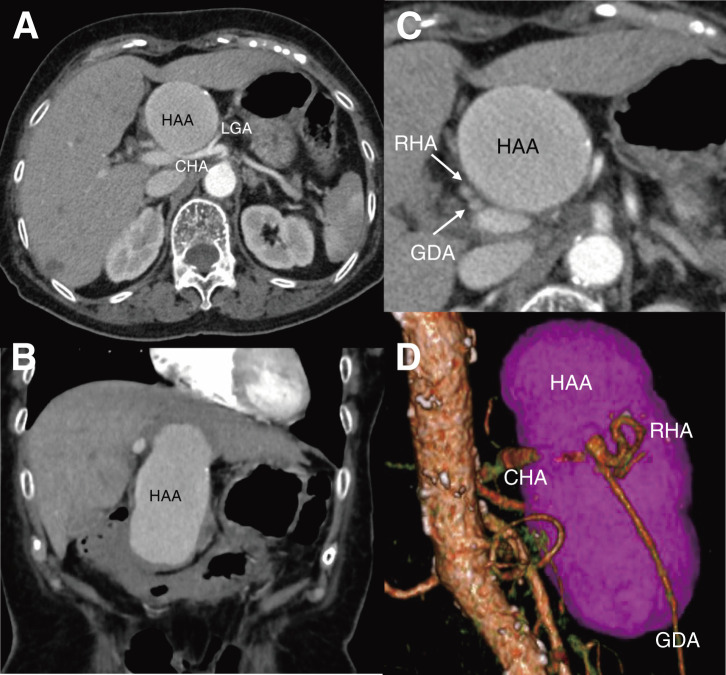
Preoperative computed tomography angiography demonstrating a large common HAA measuring 100 × 49 mm. (A) Axial view and (B) coronal view showing the aneurysm compressing the gastric antrum. (C) The aneurysm is located proximal to the GDA and RHA. (D) Three-dimensional posterior view showing a short distal landing zone from the bifurcation of the GDA and proper hepatic artery. CHA: common hepatic artery; HAA: hepatic artery aneurysm; LGA: left gastric artery; RHA: right hepatic artery; GDA: gastroduodenal artery
